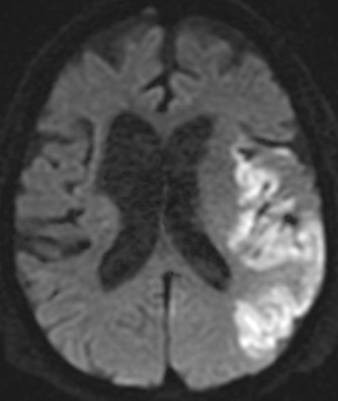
Diffusion-weighted image of the brain MRI taken on day 9 with high intensity areas on the left middle cerebral artery region.
